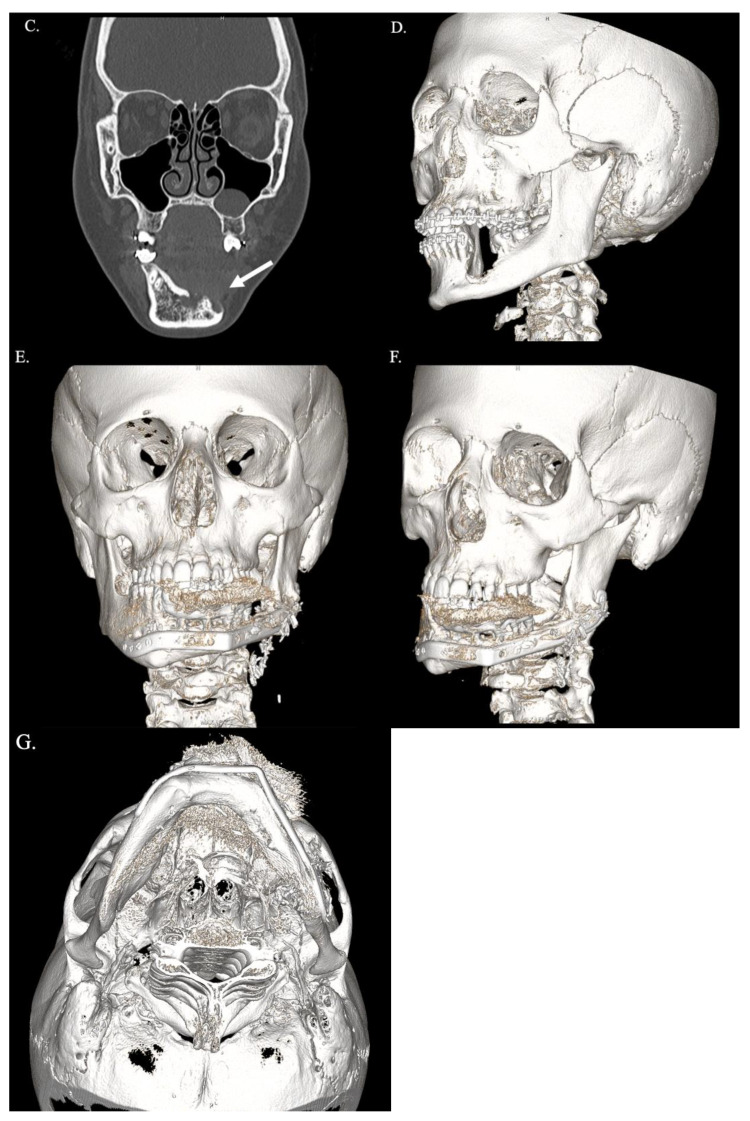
Pre-operative contrast-enhanced CT imaging of mandibular lesion. Axial CT with contrast, bone reconstruction/window (A). Axial CT with contrast, soft tissue reconstruction/window (B). Coronal CT with contrast, bone reconstruction/window (C). Three-dimensional multidetector computed tomography (MDCT) reconstruction (oblique lateral view) demonstrating osseous anatomy of mandible and involved mandibular segment (D). 3D CT scans at 2 years post-surgery (E–G). Coronal view demonstrating reconstruction place positioned as planned, dental implants and prosthesis in place (E). Three-quarter coronal view showing excellent neo-mandibular contour. (F) Axial view showing complete ossification at osteotomy sites (G). Contrast-enhanced CT does not demonstrate suspicious soft-tissue focal enhancement at periphery of mandibular tumor. White arrows indicate tumor on images (A–C).
